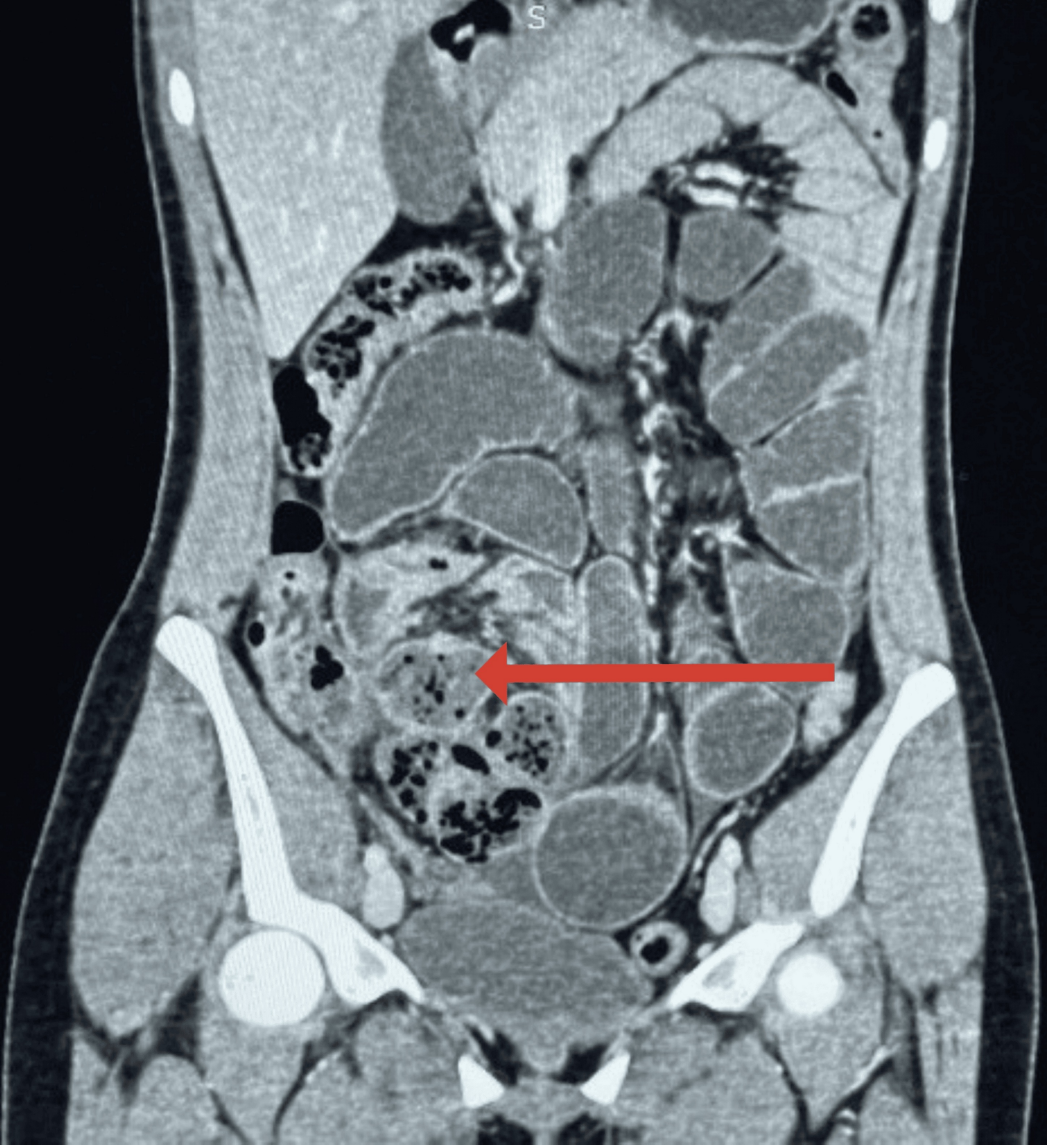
Coronal section on CECT showing similar findings, as marked by an arrow CECT: contrast-enhanced computed tomography
